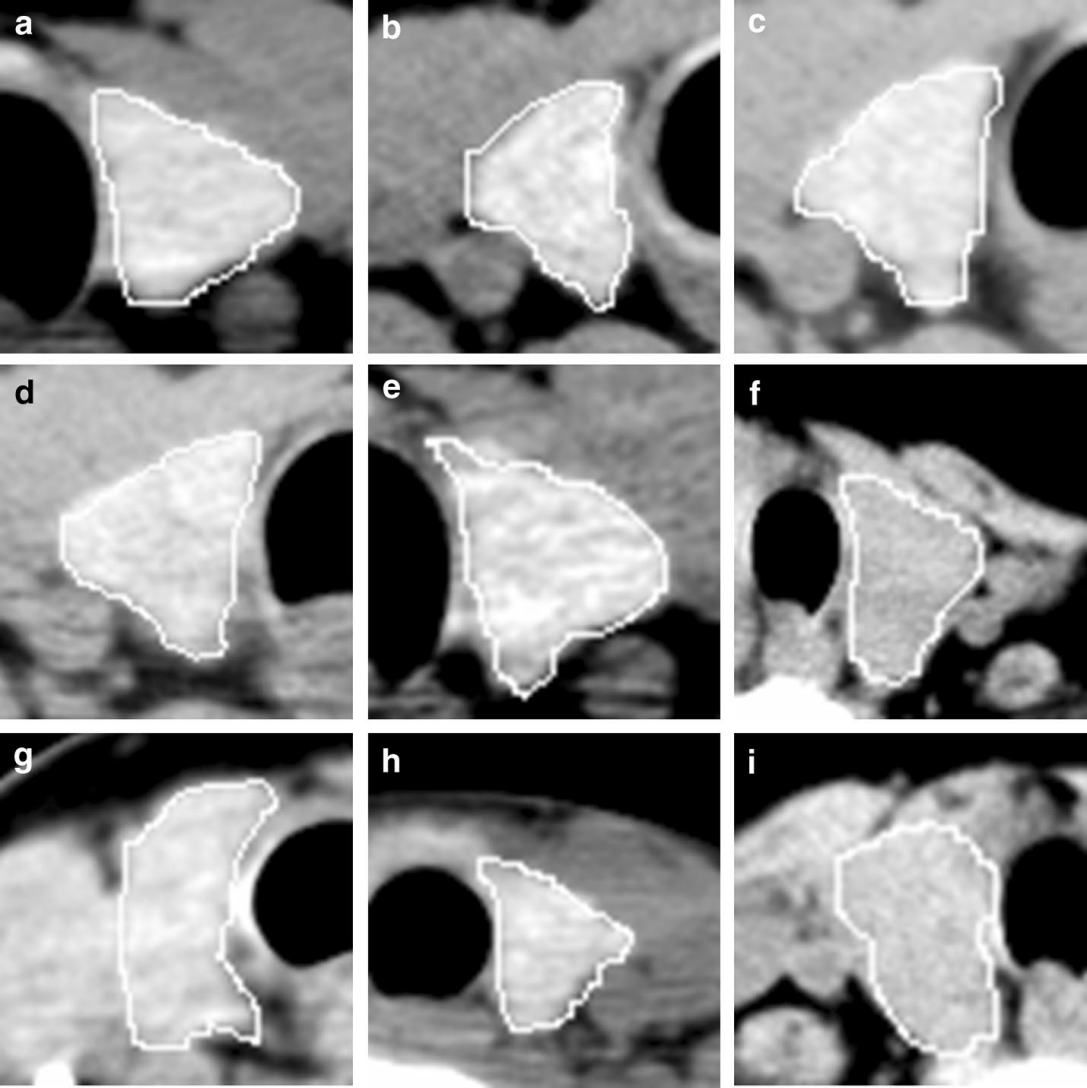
Thyroid ROIs in CT images. Images (a–i) are normal thyroid tissue from nine patients
